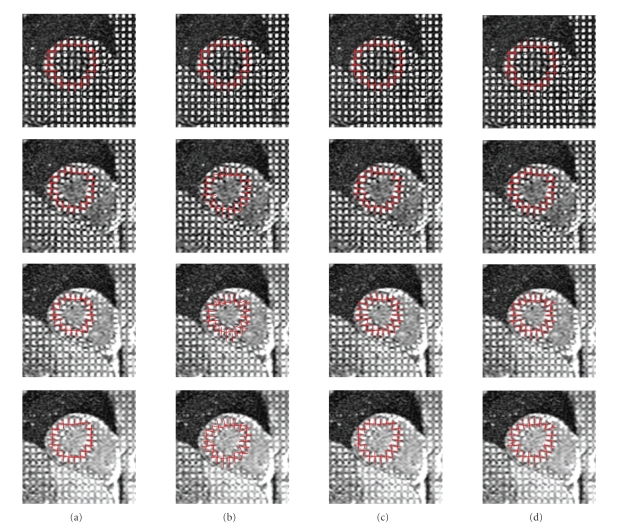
The virtual tag lines and the corresponding short-axis slices of the tagged MRI scan are shown over the cardiac cycle (first row: end diastole; third row: end
